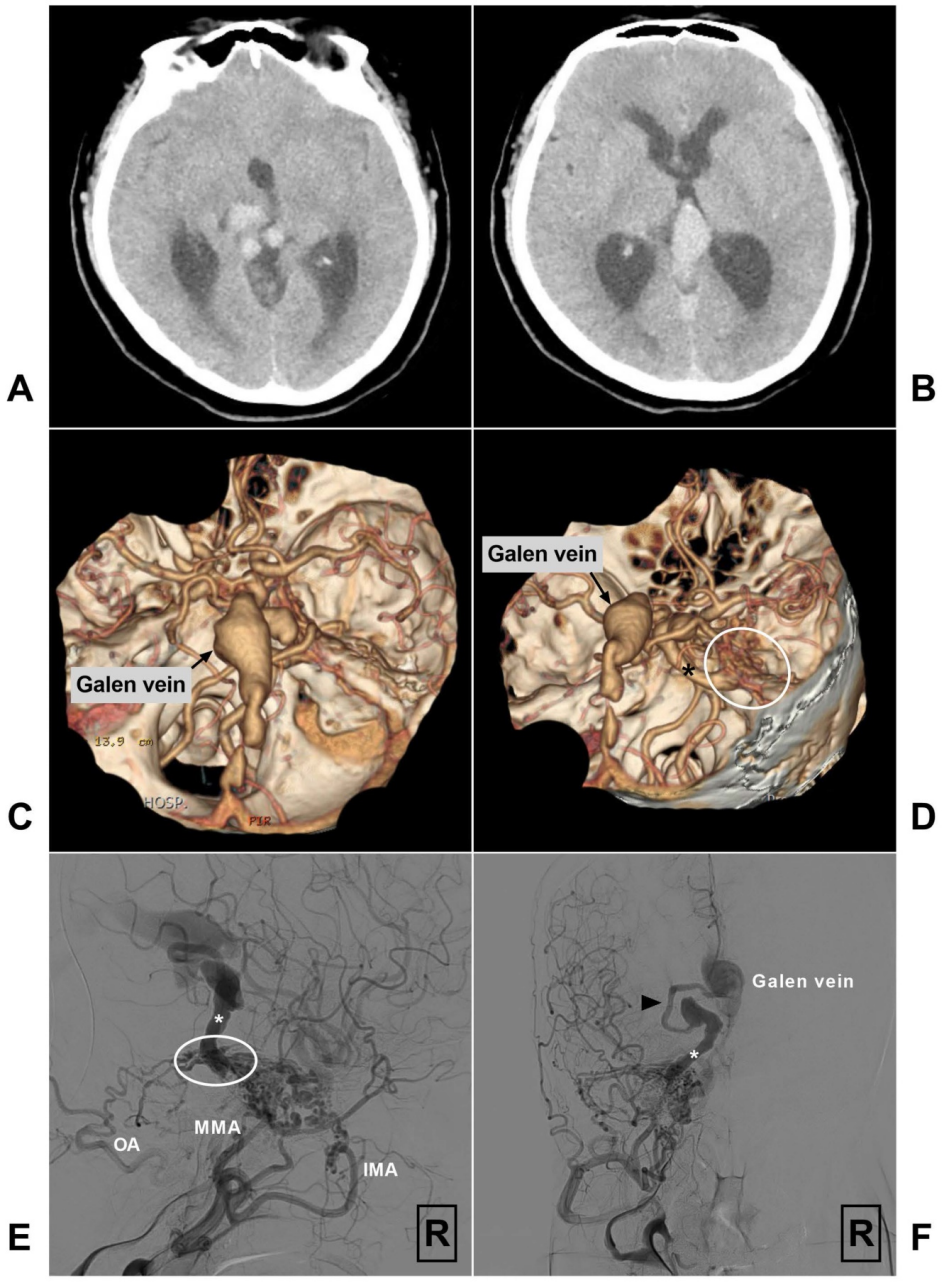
A-B, Head CT shows quadrigeminal cistern venous structure leading to obstructive hydrocephalus. C-D, CTA reveals a petroclival DAVF (encircled area) with enlarged petrosal vein (asterisk) and the Vein of Galen. E-F, Angiogram of the right ECA in lateral (E) and anteroposterior (F) views shows a SPS DAVF (encircled area) supplied by the IMA, MMA, and OA and drained retrogradely via the petrosal vein (asterisk) and pre-brainstem vein (arrow head) to the Vein of Galen. Abbreviations: CT, computed tomography; CTA, computed tomography angiography; DAVF, dural arteriovenous fistula; ECA, external carotid artery; IMA, internal maxillary artery; MMA, middle meningeal artery; OA, occipital artery, R, right; SPS: superior petrosal sinus.
